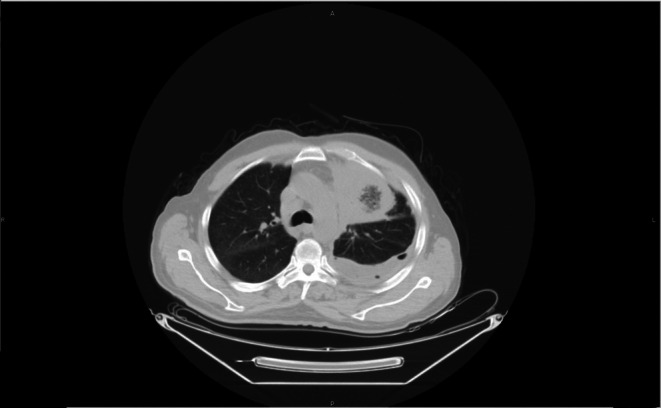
Non‐contrast CT chest showing left upper lobe consolidation with pleural effusion and underlying lung collapse.
